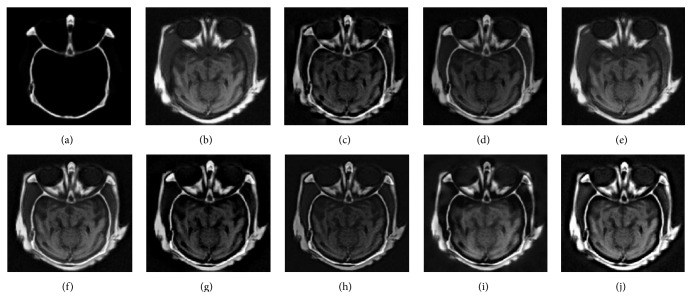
The fusion results of different fusion methods for the first set of CT and MRI images: (a), (b) Source images; fused images by (c) DWT, (d) GP, (e) PCA, (f) GF, (g) CT, (h) NSCT-1, (i) NSCT-2, and (j) the proposed method.
